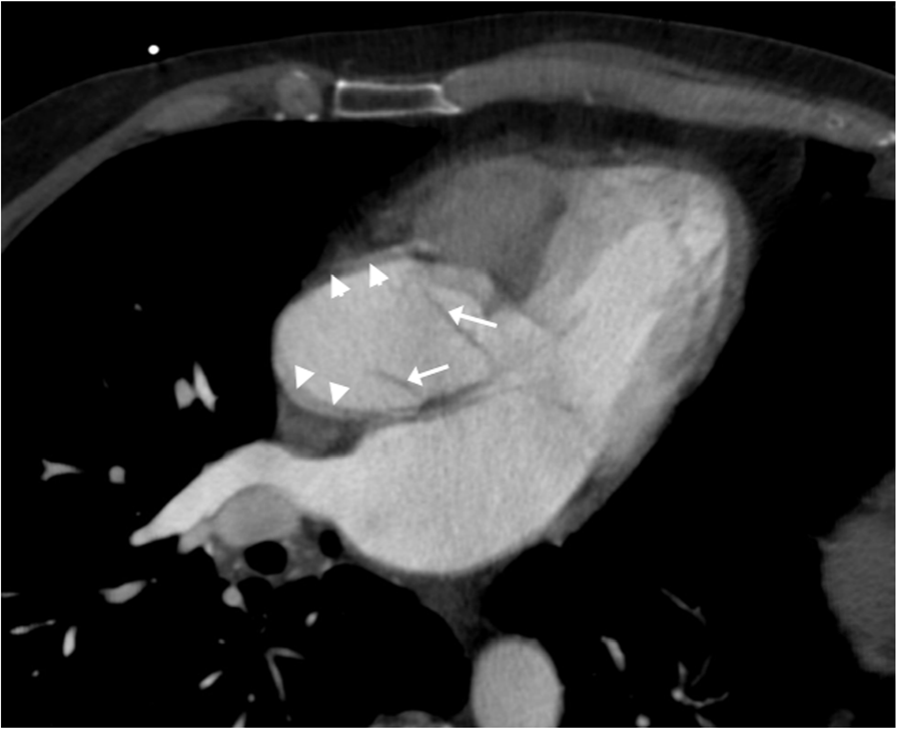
Three-dimensional reformat of the original computed tomographic angiogram. This three-dimensional reformat of the original contrast-enhanced computed tomographic scan shows the aortic root at an orientation similar to that of the transesophageal echocardiogram in Figure 2. Aortic motion artefact blurs the aortic wall (arrowheads). The lines above the aortic valve (arrows) cannot be explained by the valve leaflets and represent the dissection flap.
